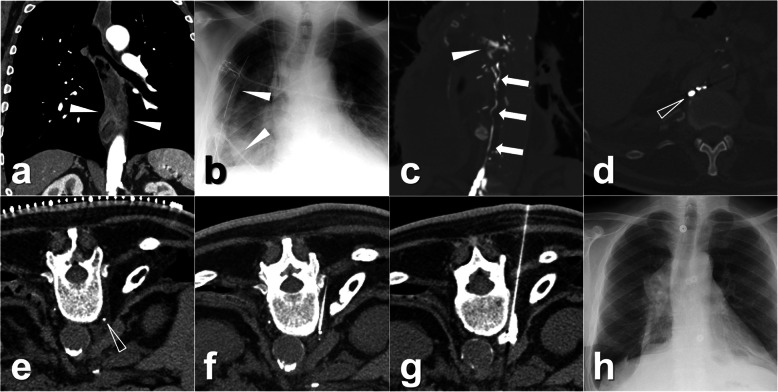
Images from a patient who was diagnosed with erosive esophagitis-induced esophageal stenosis. a Contrast-enhanced CT showed an irregular thickness of the distal esophageal wall (white arrowheads). Then the thoracic-abdominal esophagus resection plus lymphadenectomy was performed. After the surgery, the right chylothorax appeared with the average daily output of 1,130 mL. b The two drainage catheters (white arrowheads) were also observed at the ideal locations in the chest radiogram. Due to the ineffective total parenteral nutrition with a high percentage of MCTs, TL was performed, and 15.0 mL of iodinated oil was injected. c Eight hours later, CT scan showed a definite rupture (white arrowhead) of the thoracic duct (white arrows) with the extravasation to the right pleural cavity. d The distal cisterna chyli (white hollow arrowhead) was regarded as the target afferent lymphatic vessel. Because of the gradual increase of the thoracic drainage after TL, ALVS was performed 3 days later as a salvage. e In the preoperative CT scan, a small amount of residual iodinated oil could still be seen at the cisterna chyli (white hollow arrowhead). f Then, the planned puncture was achieved, and the tip was close to the cisterna chyli. g Afterwards, 1 mL if contrast agent was injected and showed an ideal distribution around the cisterna chyli. After that, 4.0 mL 95% ethanol was injected. After the procedure, the chylothorax output gradually reduced until the drainage could be removed on day 8 after ALVS. h Eleven days after ALVS, the chest radiogram showed no obvious recurrence of the chylothorax. The patient was discharged on the next day. *ALVS* Afferent lymphatic vessel sclerotherapy, *CT* Computed tomography, *MCTs* Medium-chain triglycerides, *TL* Transpedal lymphangiography
